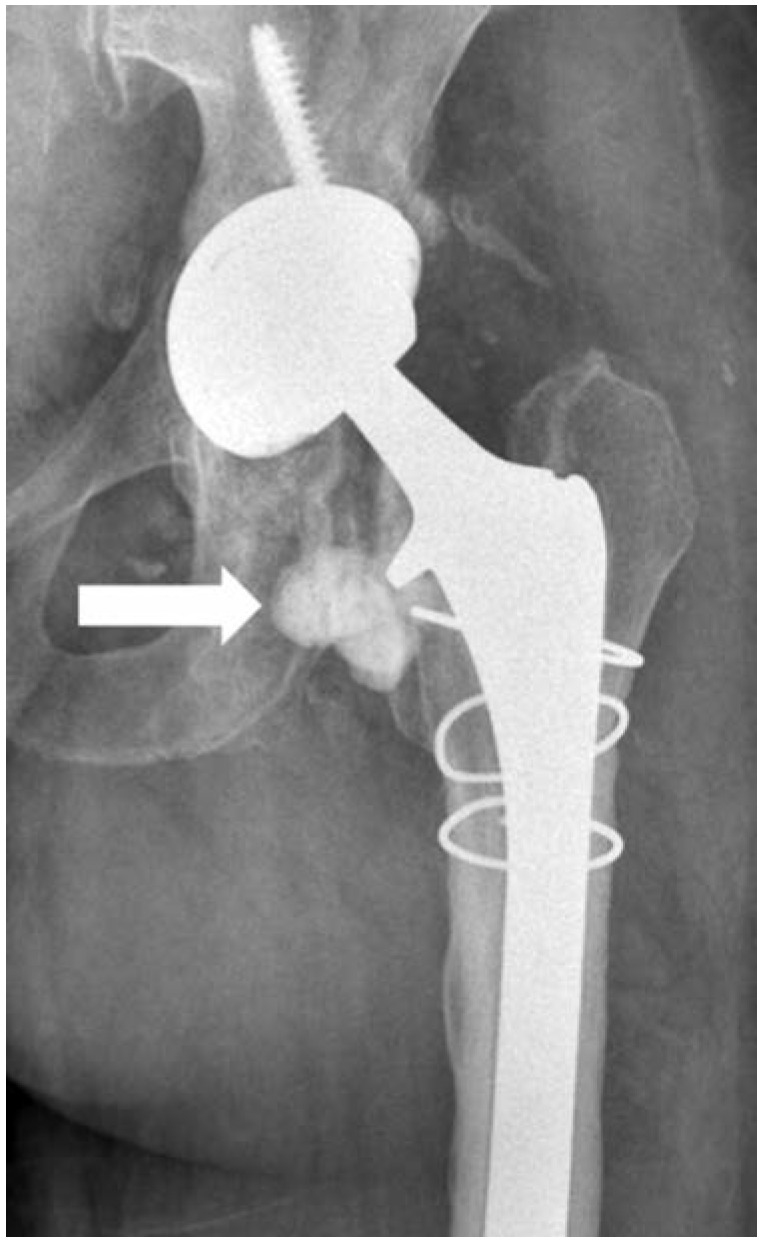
Radiograph after revision (fractured ceramic liner away from joint indicated by arrow).
